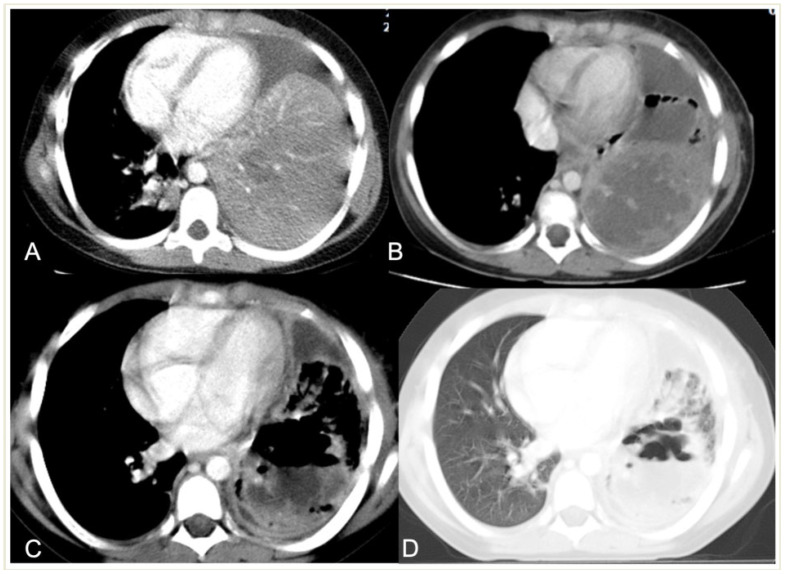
Development of necrotic and cavitary pneumonia in a 7-year-old girl. (A) There is a left basal consolidation with a central hypo-enhancing area, consistent with early necrosis. (B) Follow-up CT scan shows extensive areas of non-enhancement, consistent with extensive necrotic pneumonia. (C,D) Follow-up post-contrast scan, soft tissue, and lung window demonstrate cavitary necrosis containing multiple air–fluid levels.
